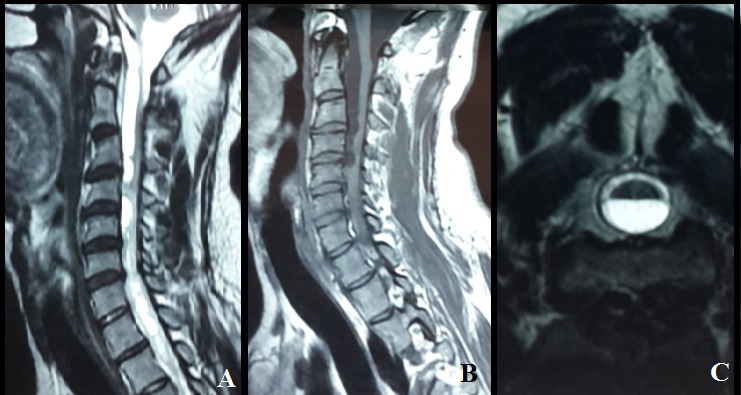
MRI of the neck shows syringomyelia cervical: A) sagittal T2; B) sagittal T1; C) axial T2
